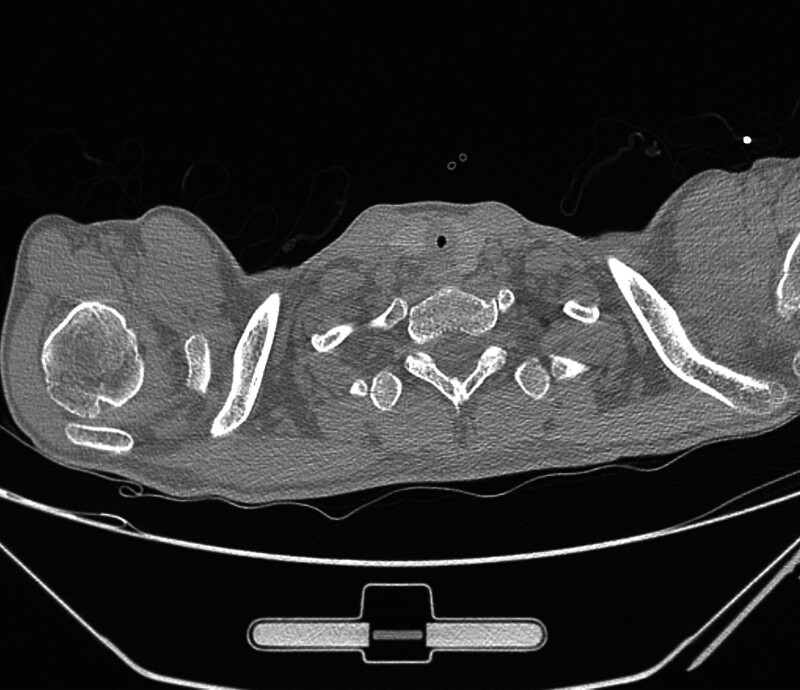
CT image of stenotic trachea at the second admission. CT = computed tomography.
